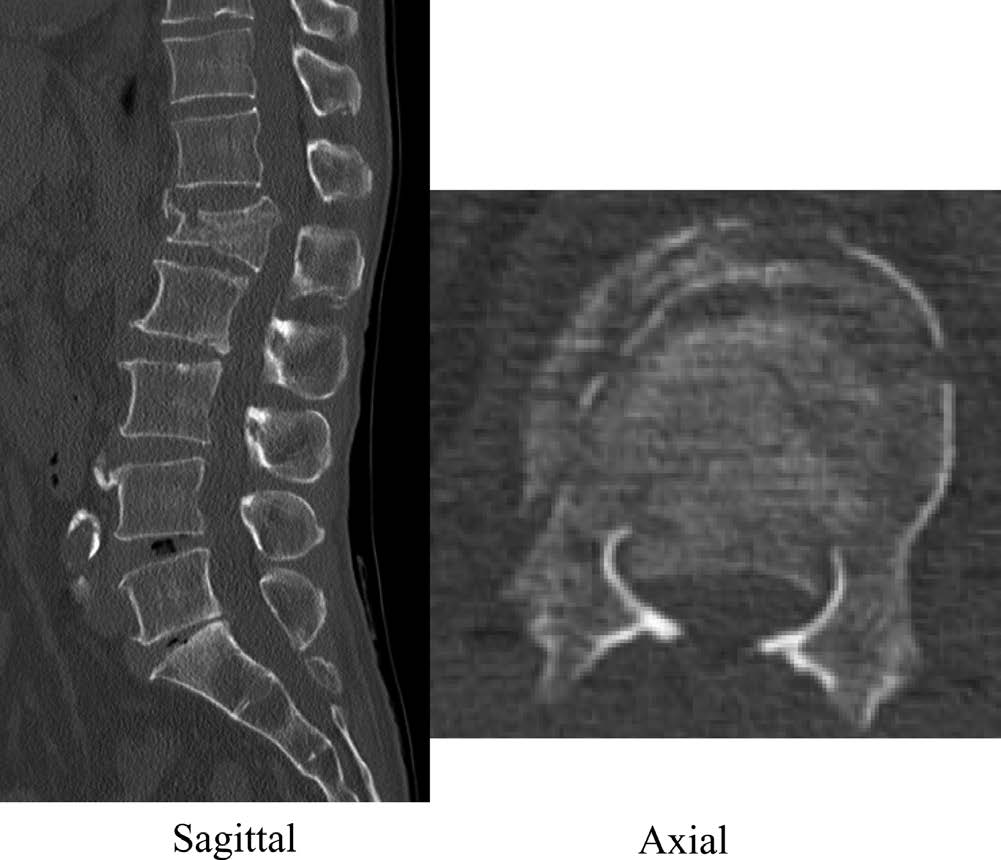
Computed tomography revealed a L1 burst fracture with a highly protruding bone fragment in the spinal canal. The bone fragment occupancy rate was approximately 50%.
